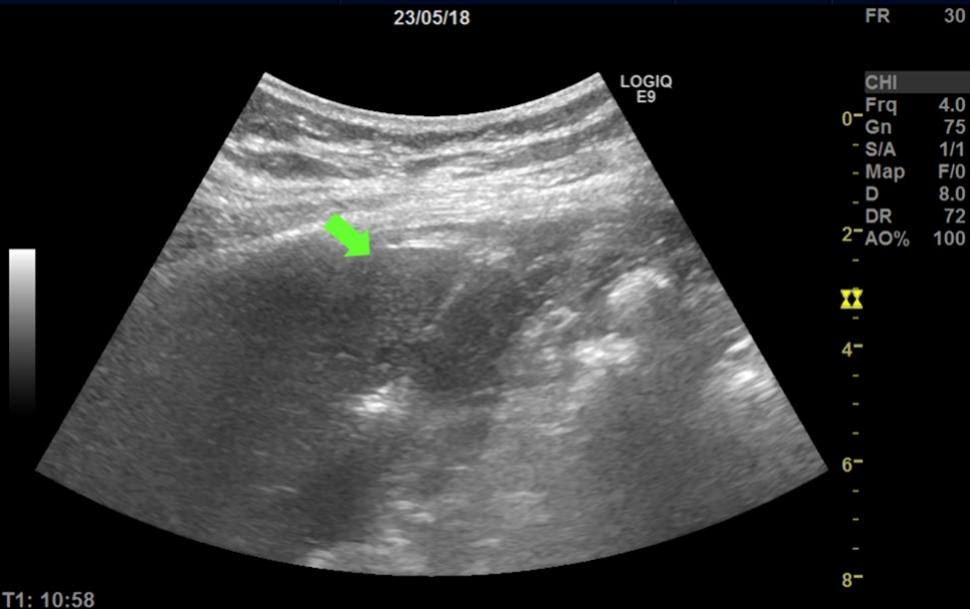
Ultrasound (US) image of the radiofrequency ablation. The image shows a hyperechoic line (needle, pointed with the arrow) inserted in splenic parenchyma
